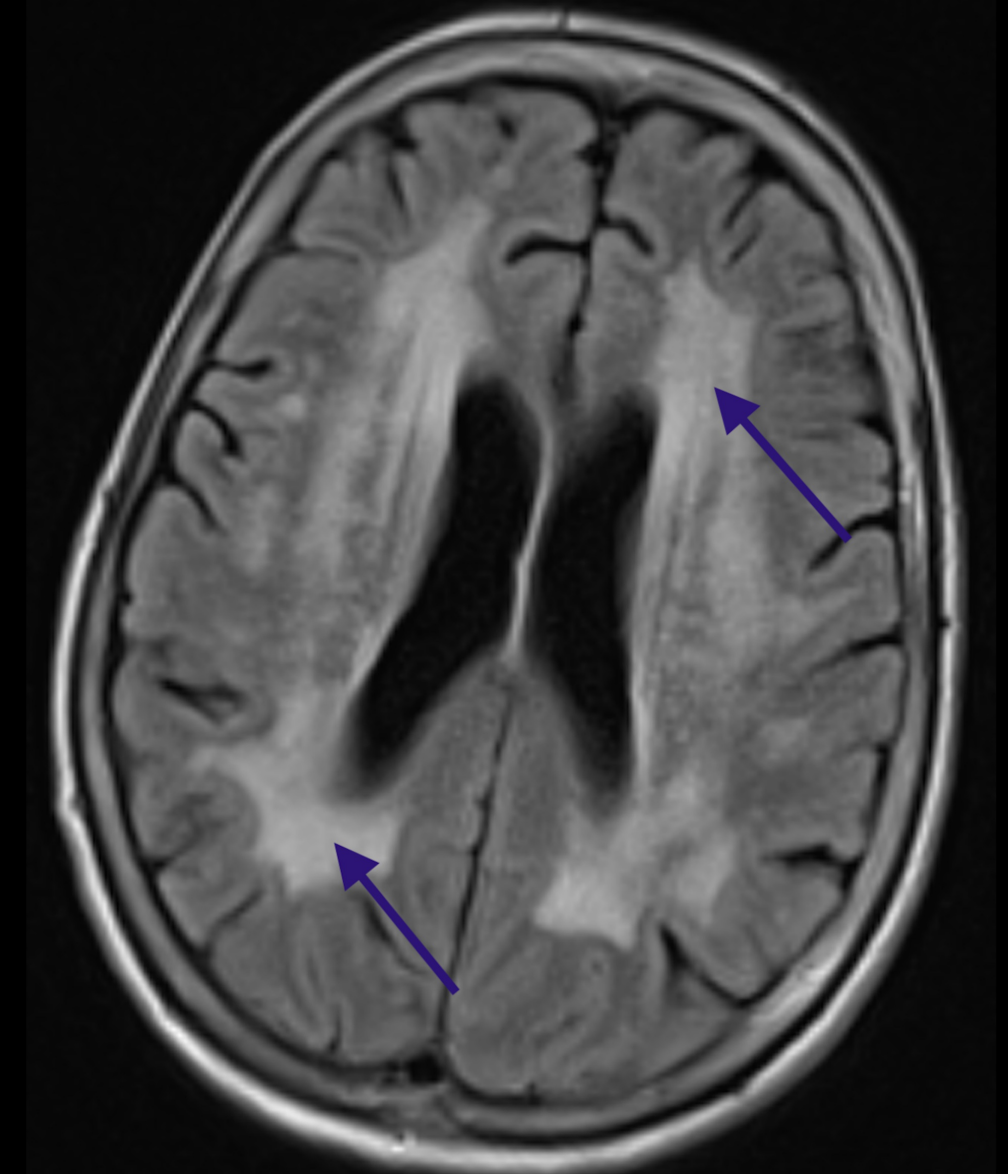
MRI of the brain: Demonstration of severe periventricular white matter disease, Binswanger's pattern (blue arrows) MRI: Magnetic Resonance Imaging
